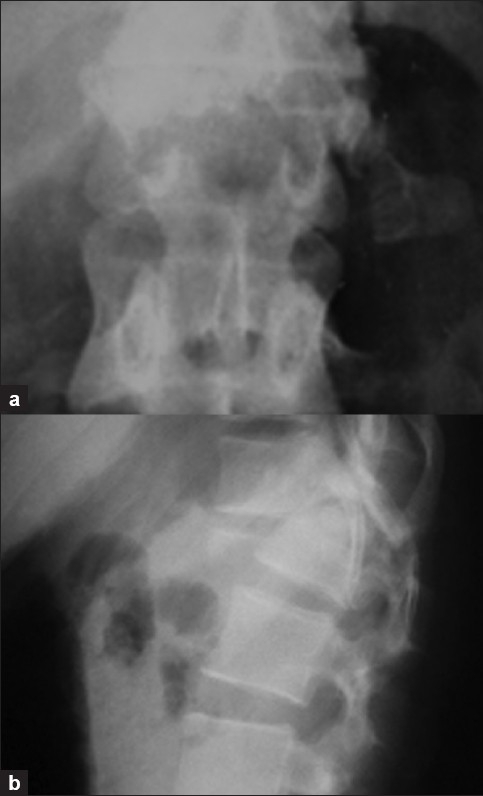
Preoperative X-ray [anteroposterior view (a) and lateral view (b)] of dorsolumbar junction showing fracture L1 vertebra
